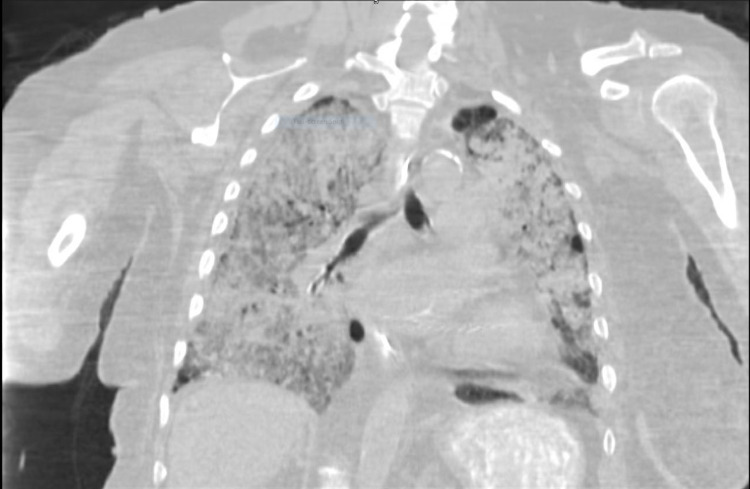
HRCT scan of our patient's chest showing the diffuse lung disease and peripheral nodules. HRCT scan = High-resolution CT scan
